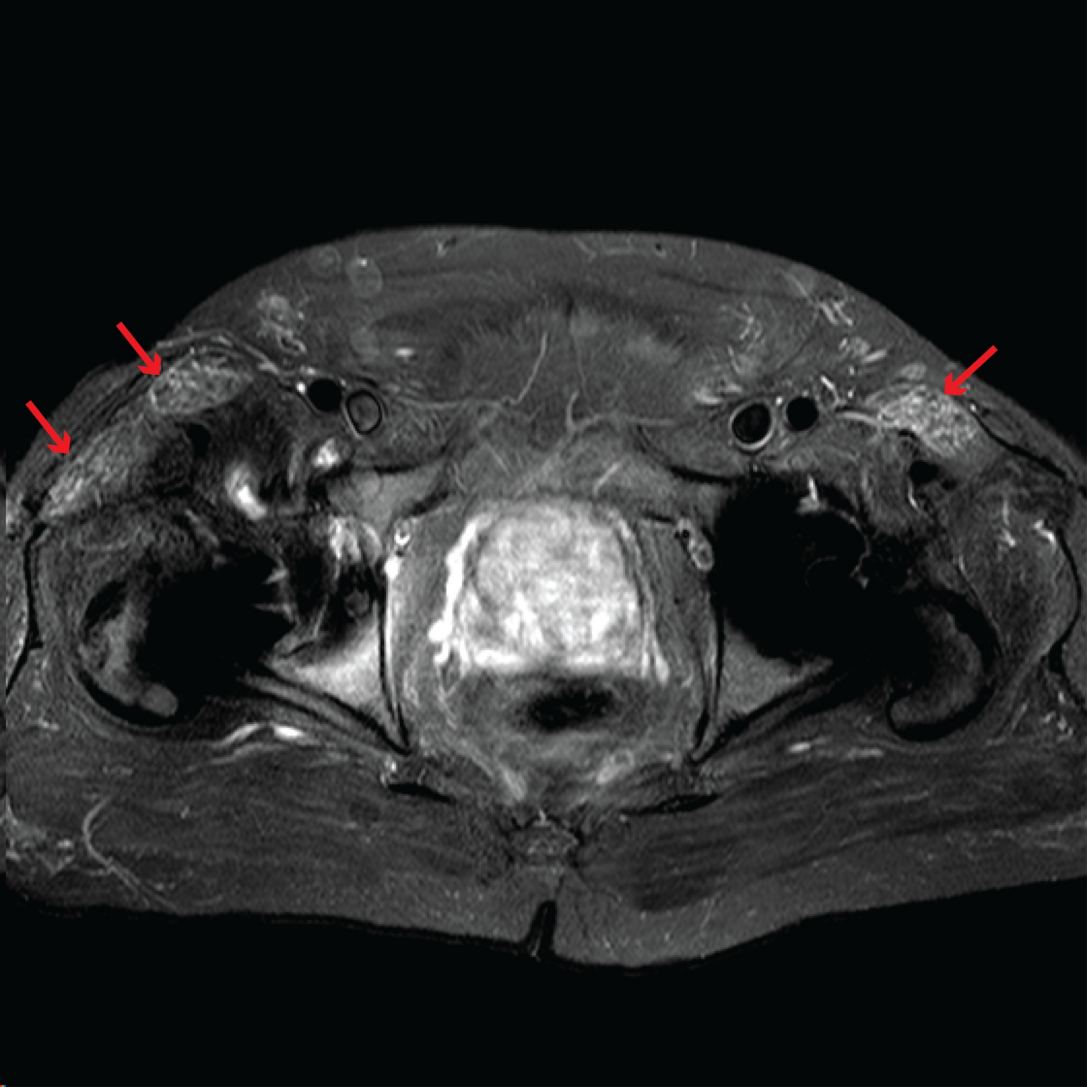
Magnetic Resonance Imaging (MRI) Pelvic MRI showed mild increased short TI inversion recovery (STIR) signal in anterior thigh muscles bilaterally, indicated by red arrows. Findings were consistent with inflammatory myopathy.
